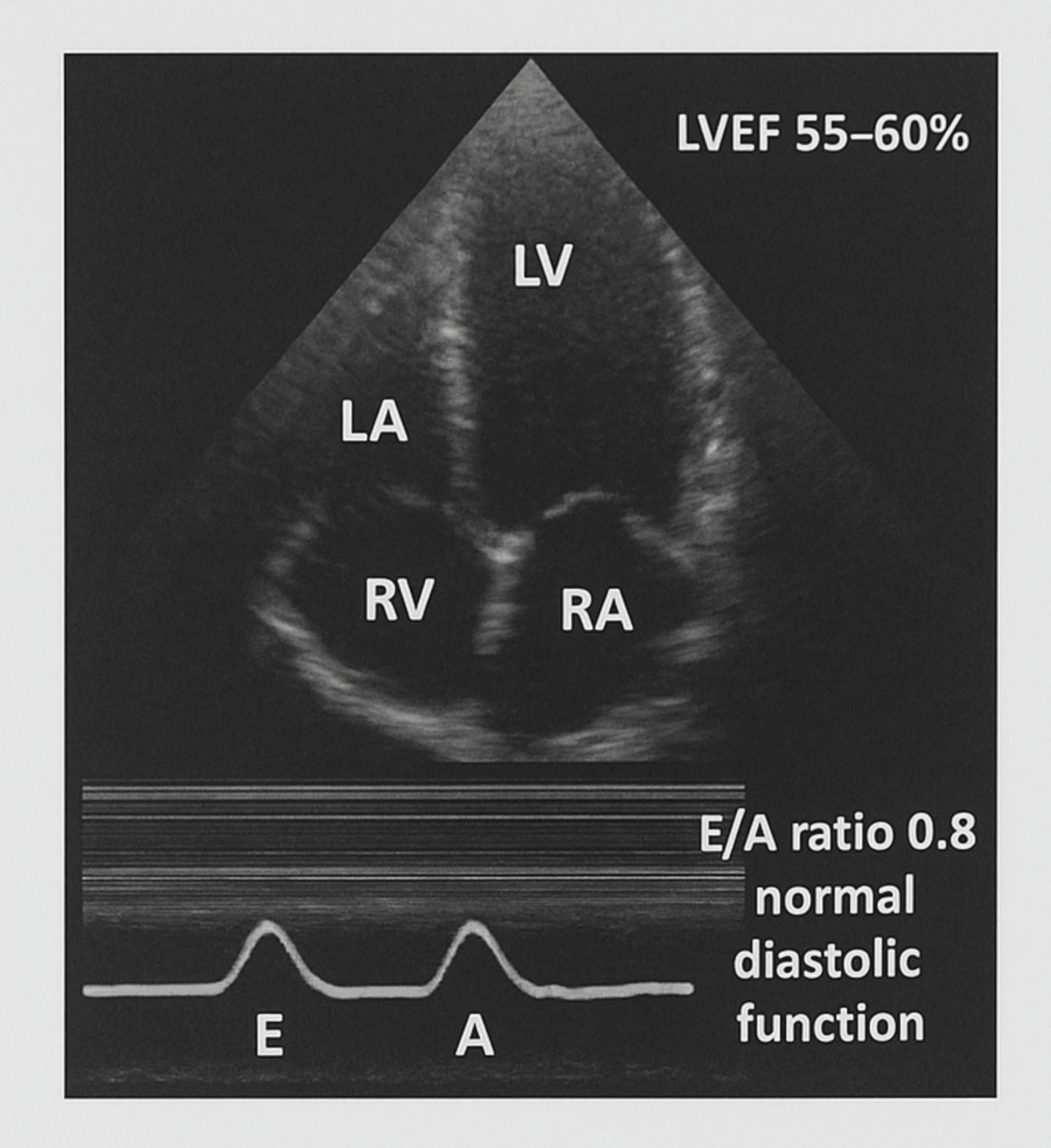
Apical four-chamber transthoracic echocardiogram The left ventricle (LV), right ventricle (RV), left atrium (LA), and right atrium (RA) are labeled. An inset M-mode tracing of mitral valve inflow demonstrates normal diastolic function (E/A ratio 0.8). The estimated left ventricular ejection fraction (LVEF) is 55–60%.
